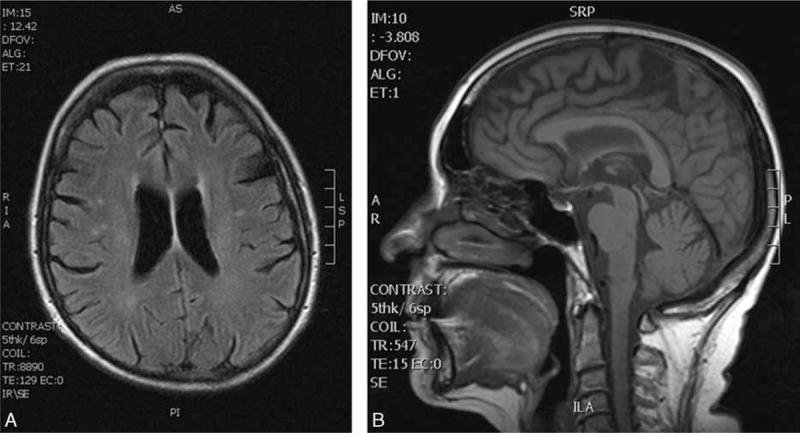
Case 2. Horizontal FLAIR sequence showing mild supratentorial changes in the white matter of both hemispheres (A). Sagittal T1-weighted MRI showing the normal shape of the brain stem (B). FLAIR = fluid-attenuated inversion recovery, MRI = magnetic resonance imaging.
